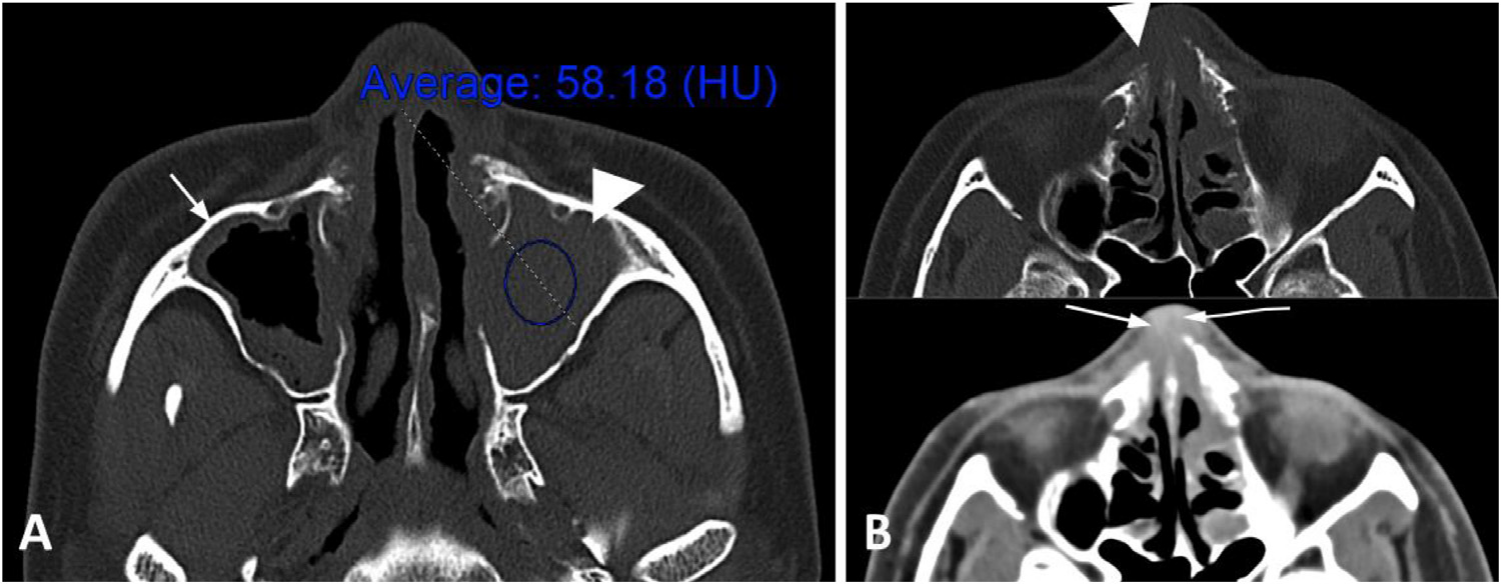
(A) Axial CT of the sinuses without contrast demonstrates complete opacification of the left maxillary sinus (white arrowhead), mucosal thickening of the right maxillary sinus (white arrow). The fluid contents of the left maxillary sinus measured average 58 HU, representing inspissated secretions. (B) Axial CT of the sinuses without contrast in bone and soft tissue window at the level of the ethmoid air cells demonstrates partial opacification of the ethmoid air cells. There is a soft tissue density along the nasal bridge (white arrow) with partial erosion and flaring of the nasal bones (white arrowhead).
